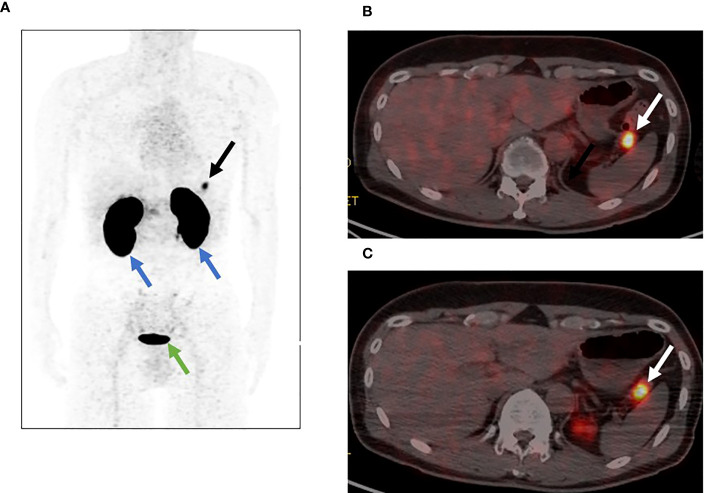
[^18^F]FB(ePEG12)12-exendin-4 PET/CT for the pancreatic tail nodule. The arrows indicate the nodule in the tail of the pancreas. (A) Maximum intensity projection of PET 1 h after administration. The black arrow indicates the nodule in the tail of the pancreas, the blue arrows indicate the kidneys, and the green arrow indicates the bladder. (B) Fusion imaging of PET/CT 1 h after administration. The white arrow indicates the nodule in the tail of the pancreas. (C) Fusion imaging of PET/CT 2 h after administration. The white arrow indicates the nodule in the tail of the pancreas.
